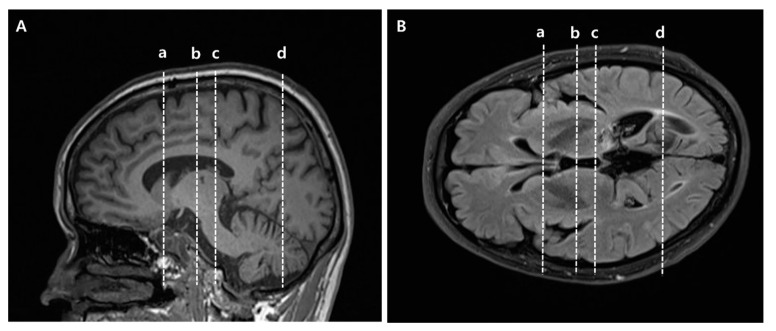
Specific anatomical locations of seed and target regions of interest (ROIs) of anterior thalamic radiation (ATR) and posterior thalamic radiation (PTR) in slice levels in brain MR images. (A) Sagittal view for ROIs, (B) Axial view for ROIs. (a) Target ROI of ATR where the frontal and temporal lobes are separated. Equivalent slice level to (a) in Supplementary Materials Figure S3. (b) Seed ROI of ATR located on the anterior part of the thalamus where the substantia nigra first appears. Equivalent slice level to (b) in Supplementary Materials Figure S3. (c) Seed ROI of PTR located on the posterior part of the thalamus, where the posterior tip of the putamen lies. Equivalent slice level to (c) in Supplementary Materials Figure S4. (d) Target ROI of PTR located below the parieto-occipital sulcus. Equivalent slice level to (d) in Supplementary Materials Figure S4.
